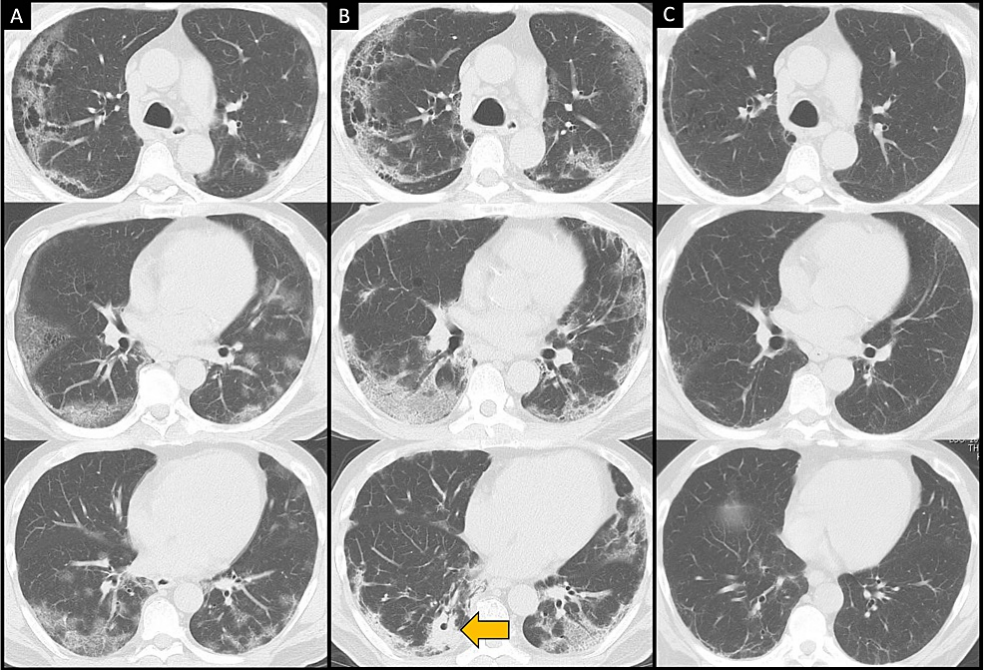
Chest computed tomography findings A) On admission, showing bilateral diffuse ground-glass opacities dominantly on the peripheral side with emphysema B) At the onset of pulmonary aspergillosis, showing consolidations with a cavity (arrow) C) After the antifungal treatment for 12 weeks, showing that the *Aspergillus* cavitary lesion with consolidation, as well as bilateral ground-glass opacities of SARS-CoV-2 pneumonia, improved
